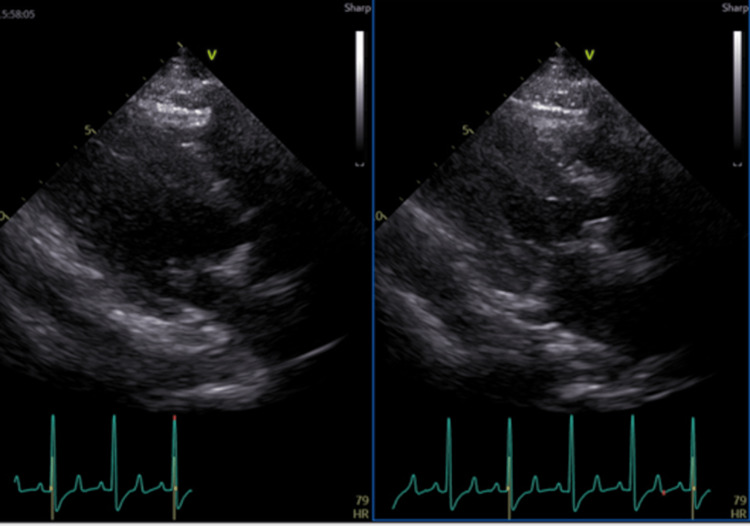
Transthoracic echocardiographic findings. TTE revealing a preserved LVEF of 79%, with no evidence of valvular dysfunction or pericardial effusion, indicative of normal cardiac function. LVEF: left ventricular ejection fraction, TTE: transthoracic echocardiography.
